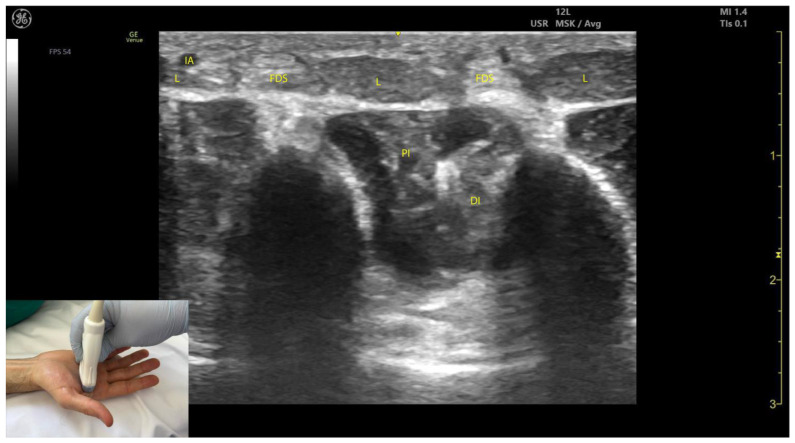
Ultrasound anatomy of the lumbricals (L) with key landmarks: L—lumbricals; PI—palmar interossei; DI—dorsal interossei; IA—interdigital artery; FDS—flexor digitorum superficialis.
